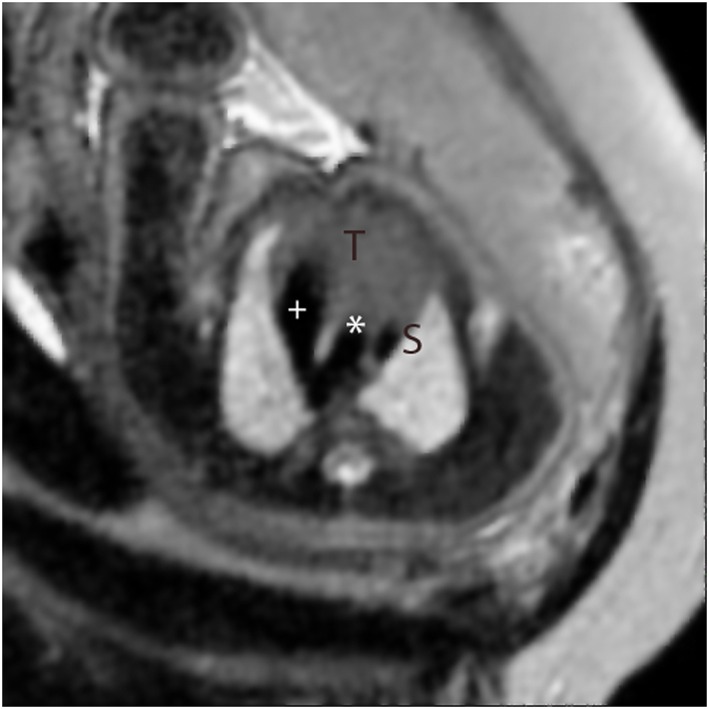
Single‐shot fast spin‐echo (SSFSE) black‐blood image in a high transverse orientation in a normal fetus. This corresponds to the standard ‘three‐vessel view’ in fetal echocardiography showing the V‐shaped connection between arterial duct (+) and the aorta (*) adjacent to the superior caval vein (S). The thymus gland is seen anteriorly (T)
